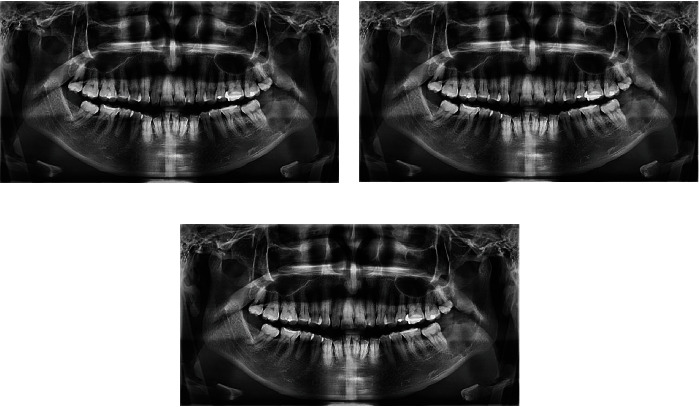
After decompression follow-up orthopantomographies. (a) 1-month follow-up. (b) 2-month follow-up. (c) 3-month follow-up.
